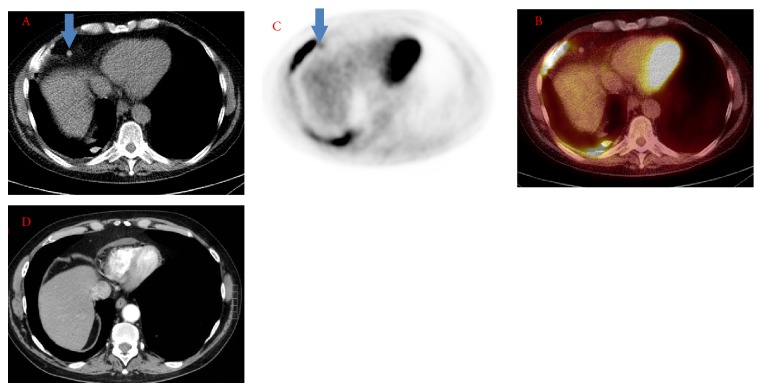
PET/CT images showing CT (A), PET (B), and fused images (C). There is an FDG avid and high-attenuation peridiaphragmatic lymph node (arrow) adjacent to a curvilinear area of FDG avid and high-attenuation pleural thickening. There is slight misregistration related to lateral excursion of the chest during respiration. A chest CT (D) performed prior to talc pleurodesis does not show any preexisting high-attenuation peridiaphragmatic lymph node.
